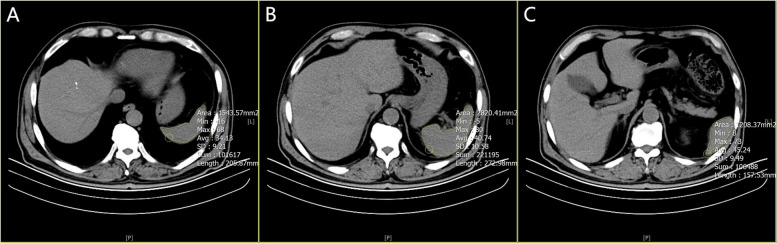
Abdominal CT scan of a colorectal cancer patient with spleen density. The CT value of spleen at the (A) upper pole level, (B) the hilum level, and the (C) lower pole level was 34.13, 40.74, and 45.24 HU, respectively
